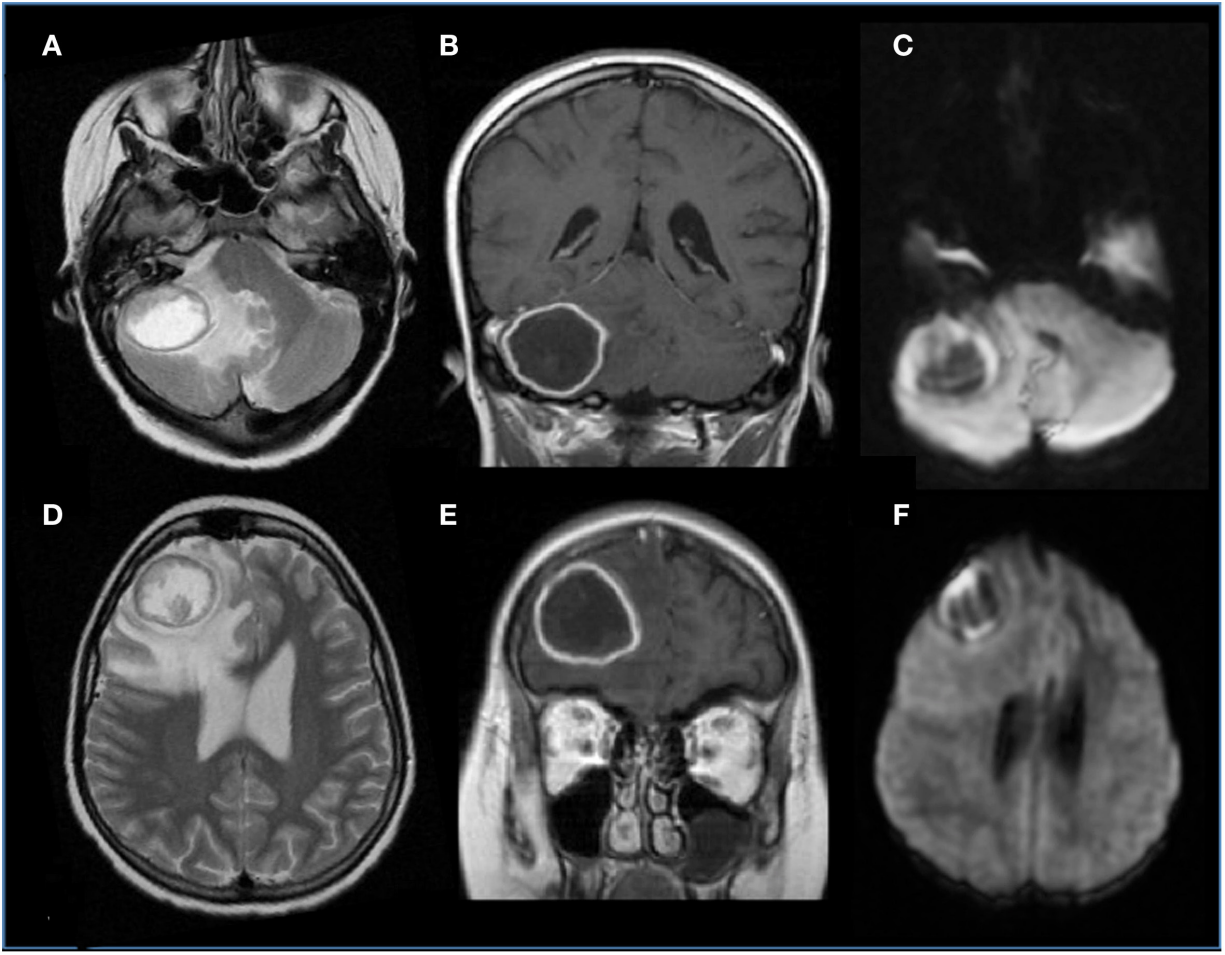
Brain aspergillosis in a 15-year-old girl with acute lymphoblastic leukemia. (A,B,D,E) Axial T2 and coronal post-gadolinium T1-weighted images, respectively, showed ring-enhancing lesions with mass effect, surrounded by abundant edema, on the right side, within the frontal lobe and cerebellar hemisphere. (C,F) Diffusion study reveals marginal hyperintensities representing peculiar intracavitary fungal hyphae projections.
